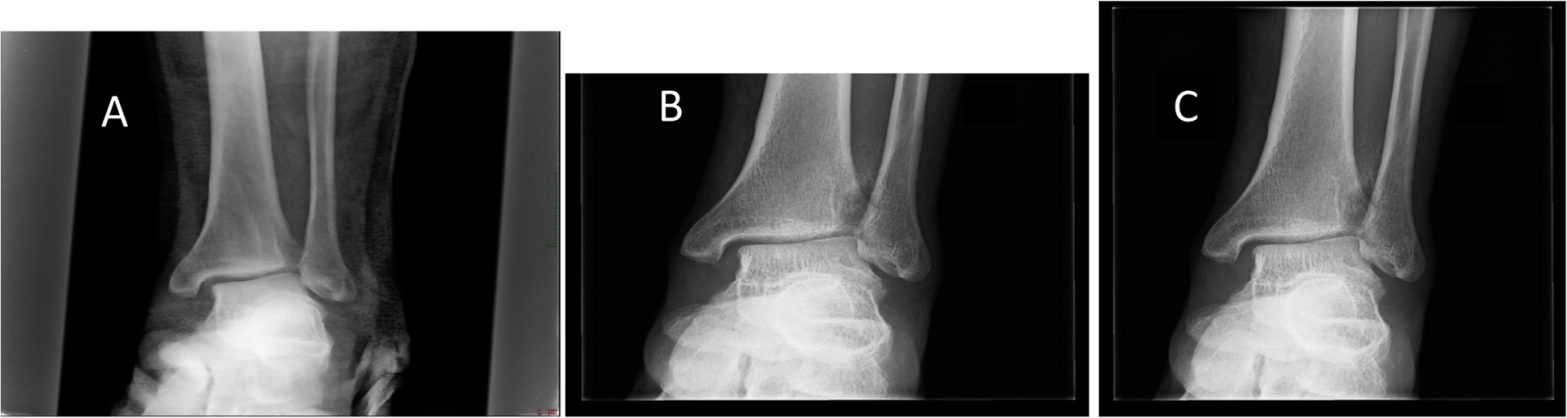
An antero-posterior radiographic view of our patient’s left ankle immediately (a), 6 (b), and 10 (c) weeks following his injury. Medial and superior tibio-talar space widening is evident on radiograph (a). Note the gradually appearing radio-opaque syndesmotic appearance (radiograph b and c)
